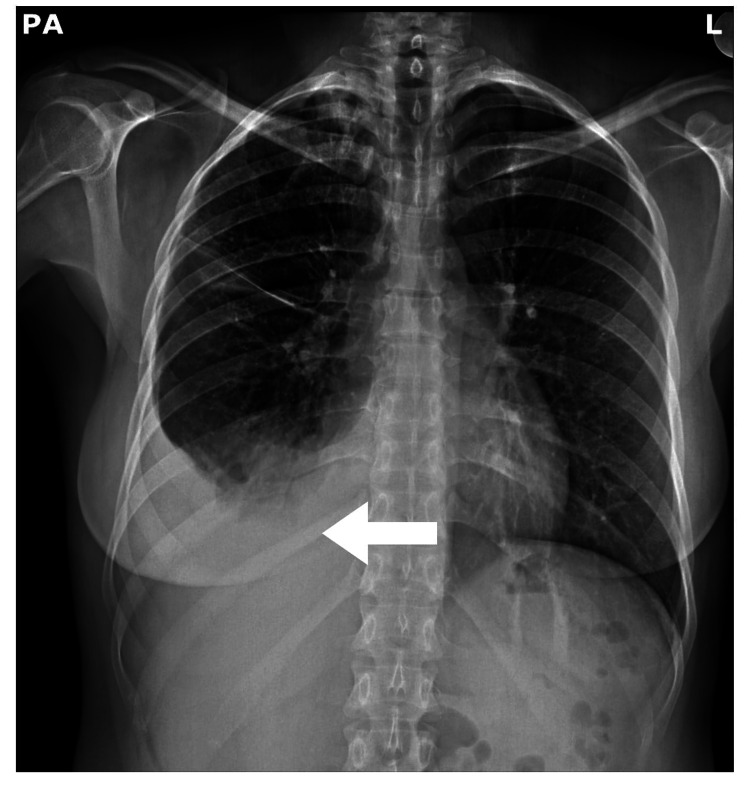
CXR PA Plain chest radiograph PA view. Arrow showing right lower lobe consolidation and right mild pleural effusion CXR: chest X-ray; PA: posteroanterior
